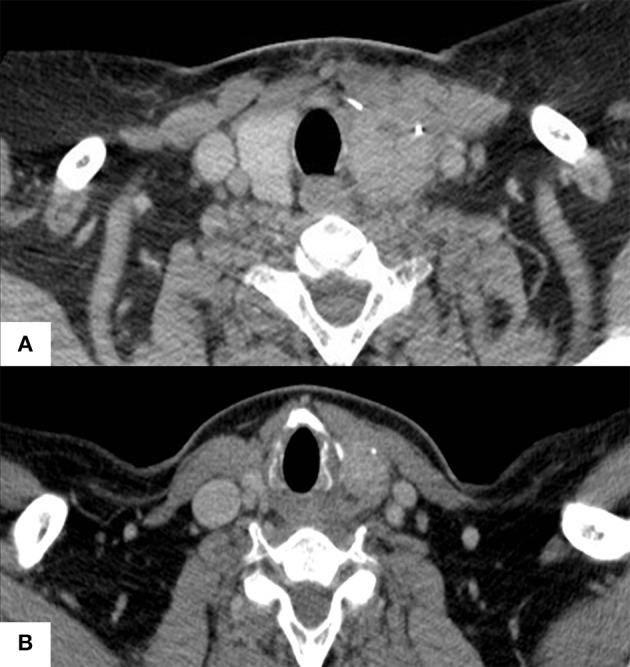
Thyroid CT scans pre (A) and after 6 months (B) of neoadjuvant sorafenib therapy.
